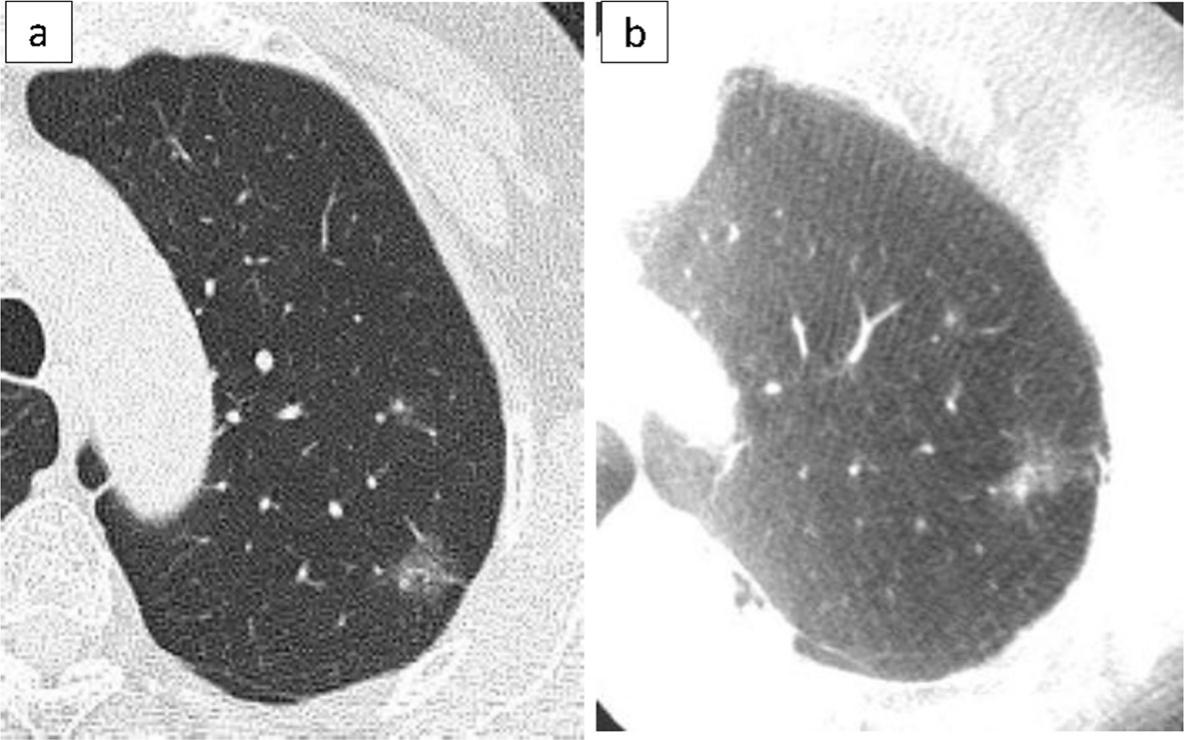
A GGN with a solid part. Preoperative CT shows a GGN, 14 mm in diameter, with a small solid part in the left upper lobe (a). The O-arm shows the same finding as the preoperative CT (b).
